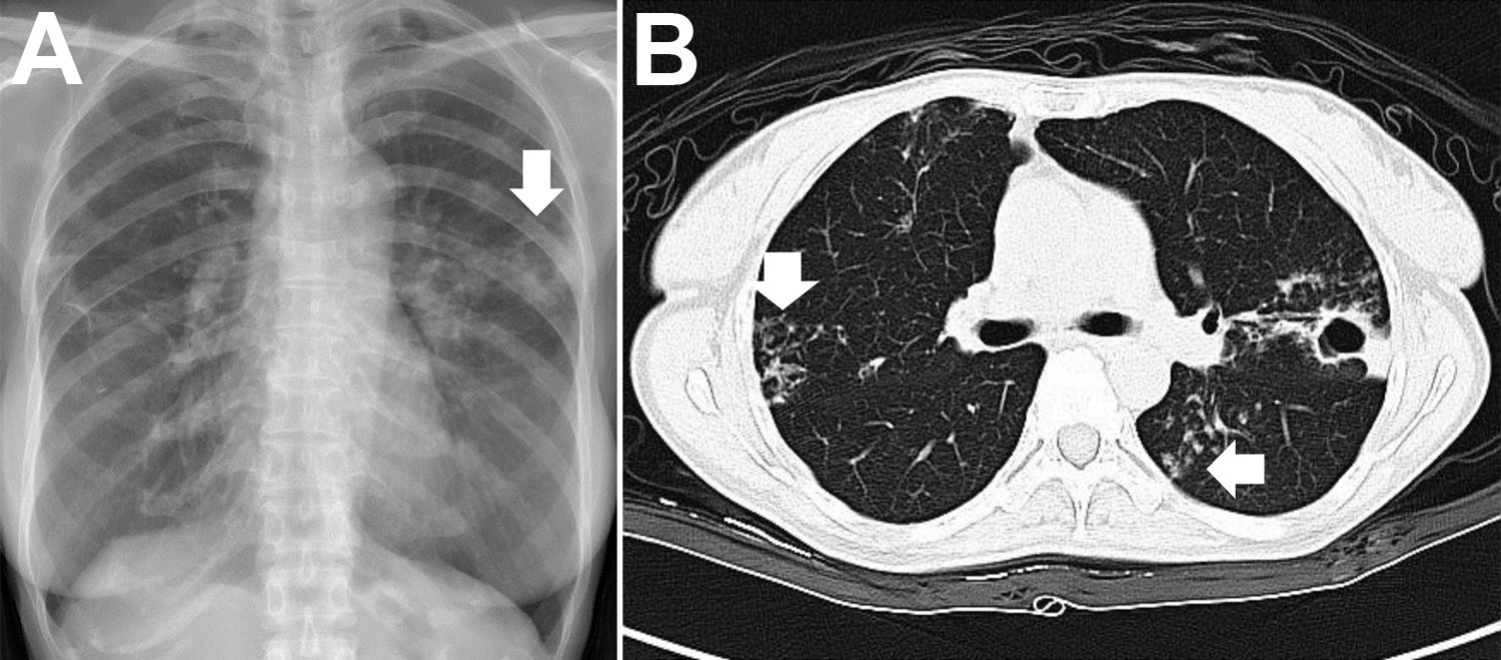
Chest radiograph (A) and computed tomography scan (B) images for a patient with pulmonary disease due to *Mycobacterium abscessus* subsp. a*bscessus*. A) The arrow indicates a cavity with surrounding consolidation over the left upper lung. B) Vertical arrow indicates bronchiectasis; horizontal arrow indicates nodules.
